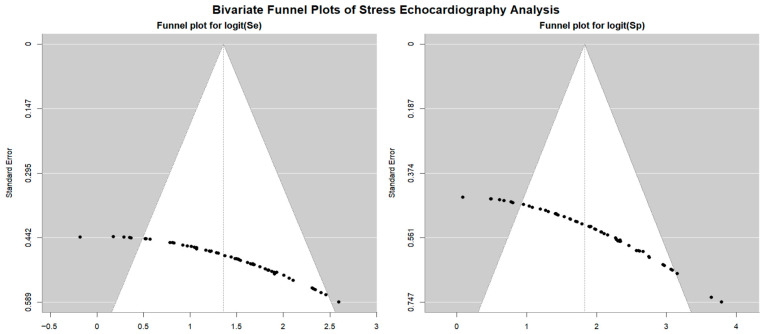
Begg’s funnel plot for the assessment of publication bias in studies evaluating sensitivity and specificity of SE for detecting obstructive CAD. CAD, coronary artery disease; SE, stress echocardiography.
